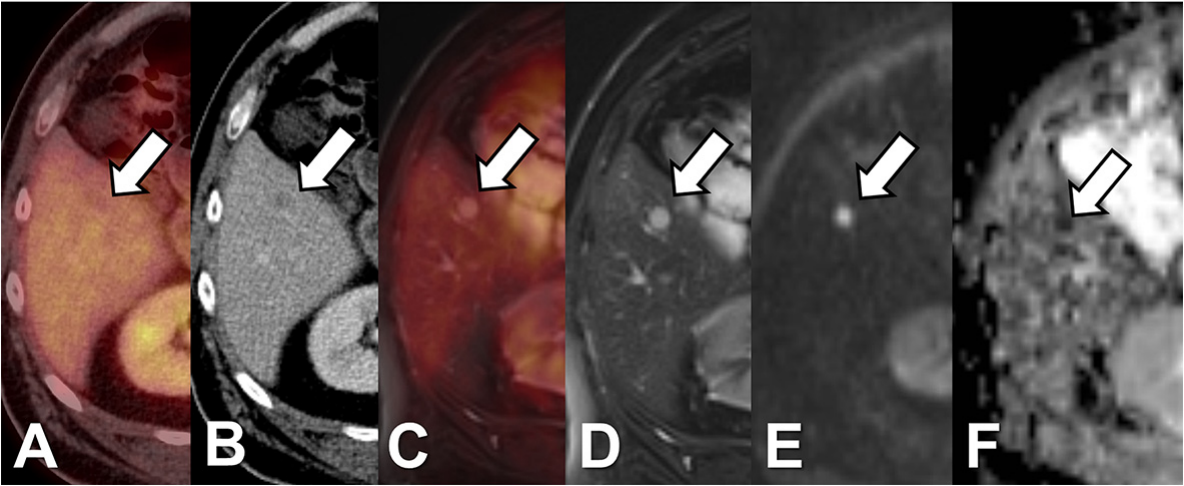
Patient with uveal melanoma. cePET/CT (A+B) shows a hypodense liver lesion of 10 mm in diameter without elevated tracer uptake which is therefore rated as indeterminate. In PET/MRI (C-F) the lesion is hyperintense in T2w TSE (D) and shows signs of restricted diffusion (E: b1000; F: ADC map). Therefore, based on PET/MRI the lesion is rated as metastasis. Based on T2w imaging additional 4 metastases are visualized in PET/MRI in the contralateral lobe.
